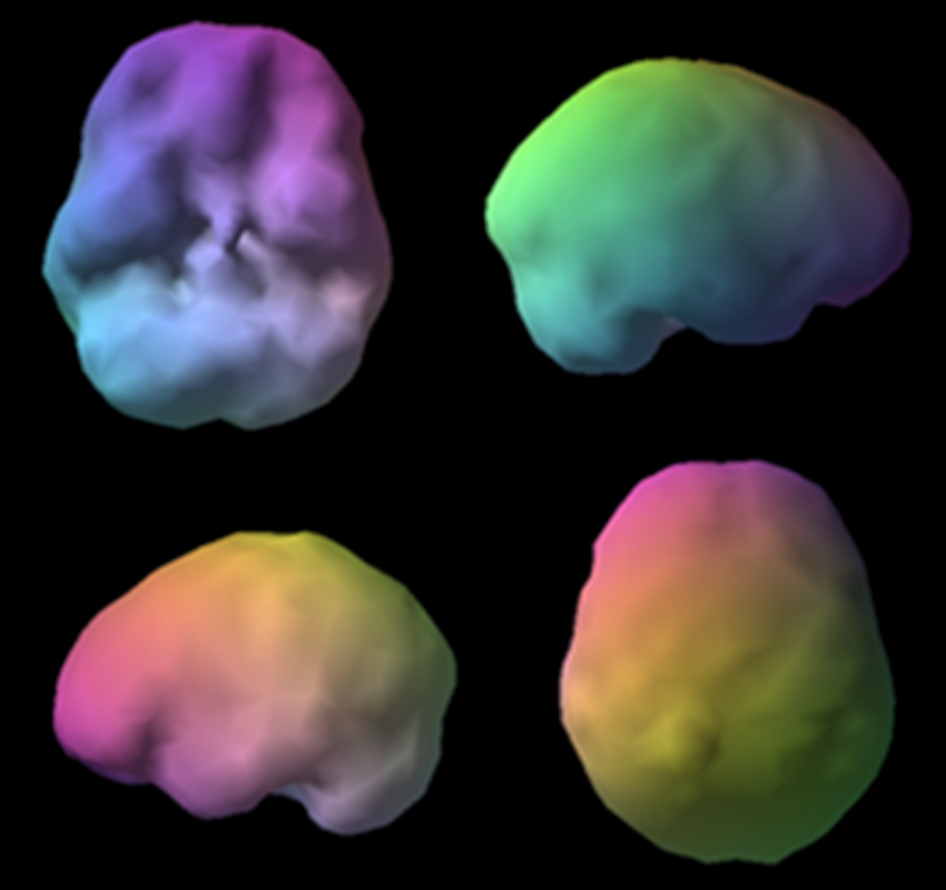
Normal surface rendering. A healthy 3D surface rendering of SPECT information, looking at the top 45% of brain perfusion. A healthy scan shows full, even, symmetrical perfusion.
